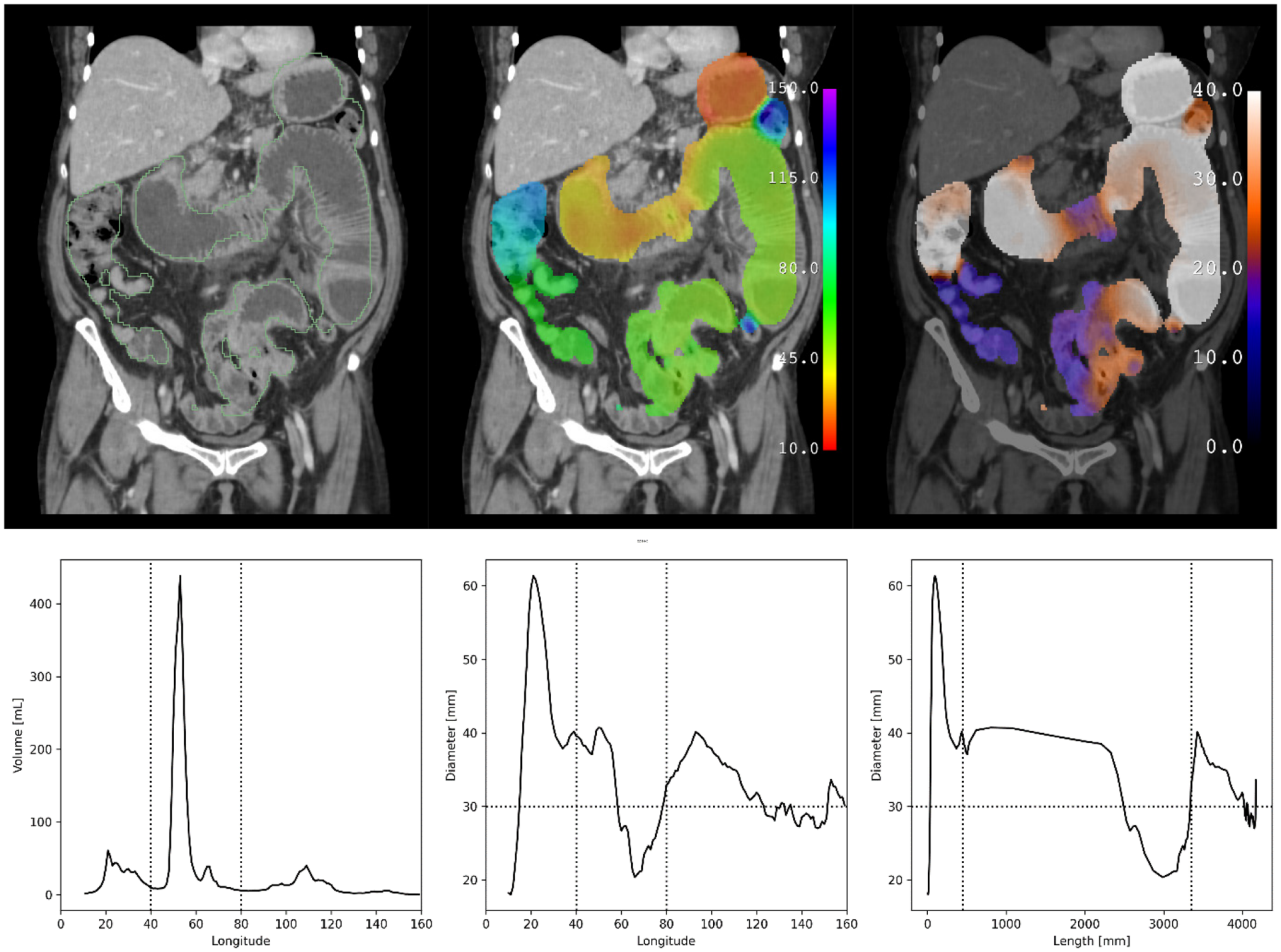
Test subject with bowel obstruction. Segmentation (green outline), longitude (rainbow), and diameter (hot metal blue) predictions superimposed on a coronal slice of a CT scan of a subject from the test data set with bowel obstruction. Graphical representations of volume and diameter (averaged over 1 unit intervals of longitude) versus longitude and length are shown for the same subject. Vertical dotted lines indicate the ligament of Treitz and ileocecal valve. Horizontal dotted line indicates the threshold for small bowel obstruction. Small bowel dilation and a transition point are present are present in this subject, as seen in the graph of diameter versus length. Several loops of nondilated distal ileum are noted to be erroneously excluded from the segmentation
